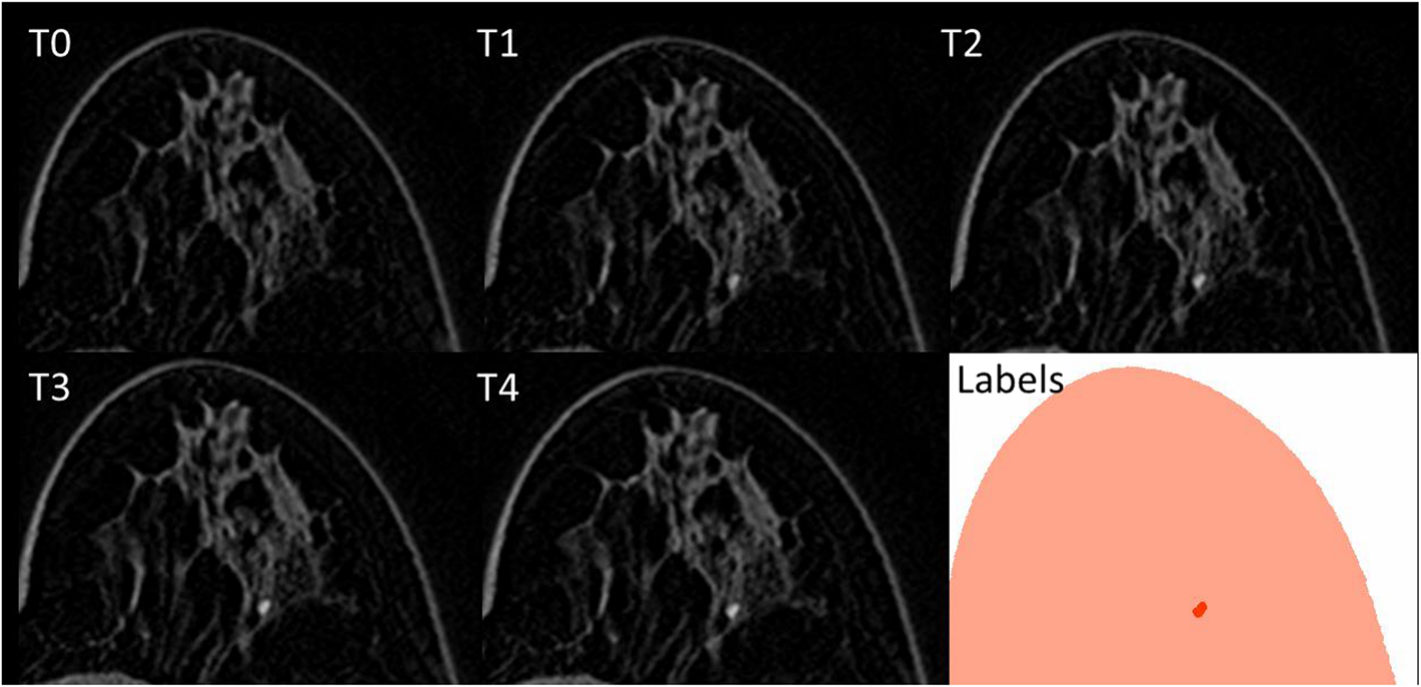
Breast magnetic resonance imaging showing in T0 the first (unenhanced) image and from T1 to T4 the contrast-enhanced images, where the wash-in and wash-out phenomena give information about the malignant or benign nature of the lesion. In the last image (“labels”), the segmented focus is coloured in red while normal breast tissues are coloured in pink
